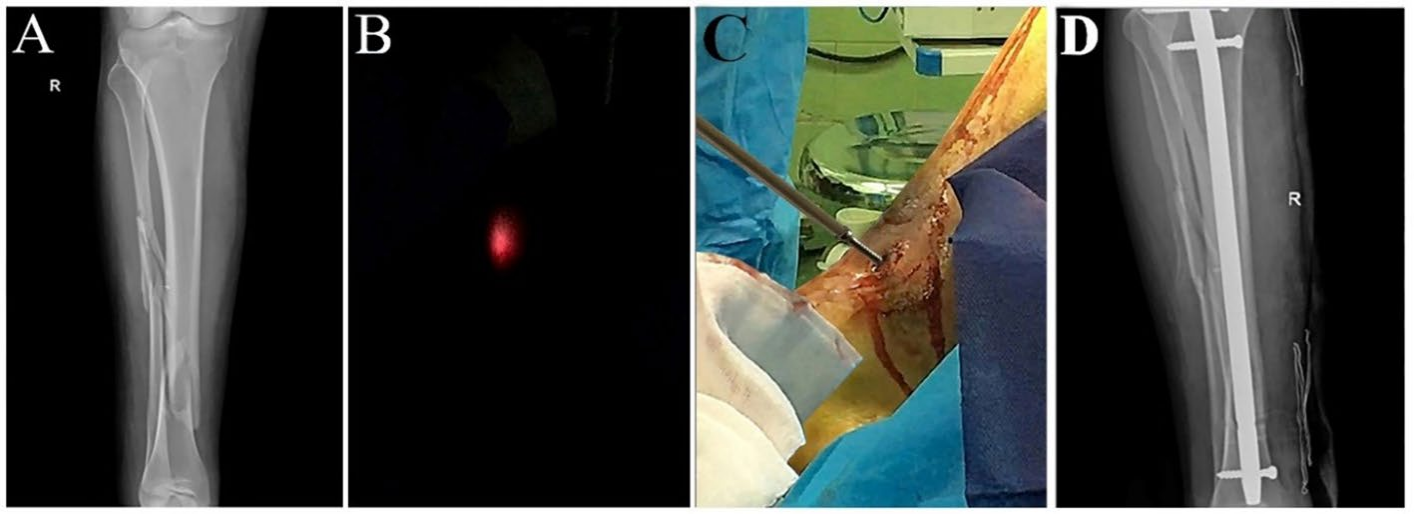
Interlocking of the intramedullary nail by the laser system, (A) X-ray image of the patient's right foot before the surgery, (B) Finding drilling position by the IMN holes laser indicator in the dark surgery room, (C) Interlocking the IMN, (D) The X-ray image of the patient's right foot one day after intramedullary nailing.
